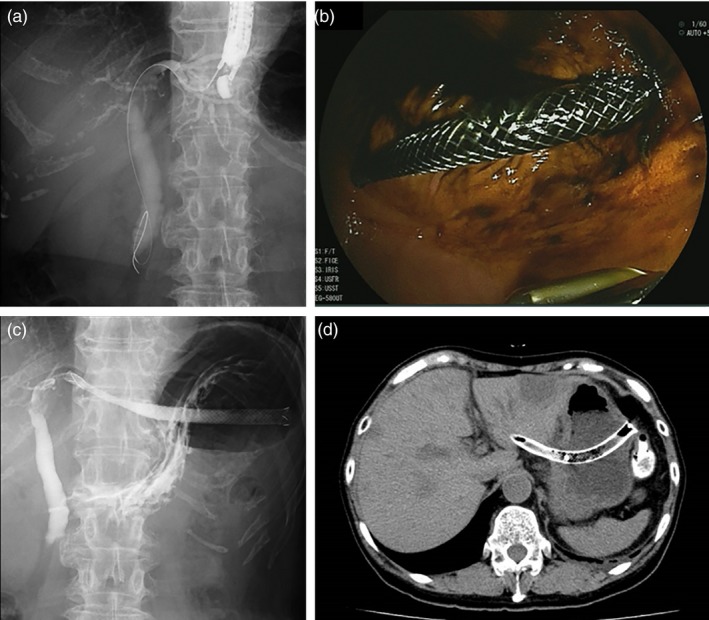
EUS‐guided hepaticogastrostomy (EUS‐HGS). (a) Fluoroscopy of EUS‐guided puncture of the left intrahepatic bile duct. (b) Endoscopic view after EUS‐HGS. (c) Fluoroscopy after EUS‐HGS. (d) CT image of the EUS‐HGS stent
